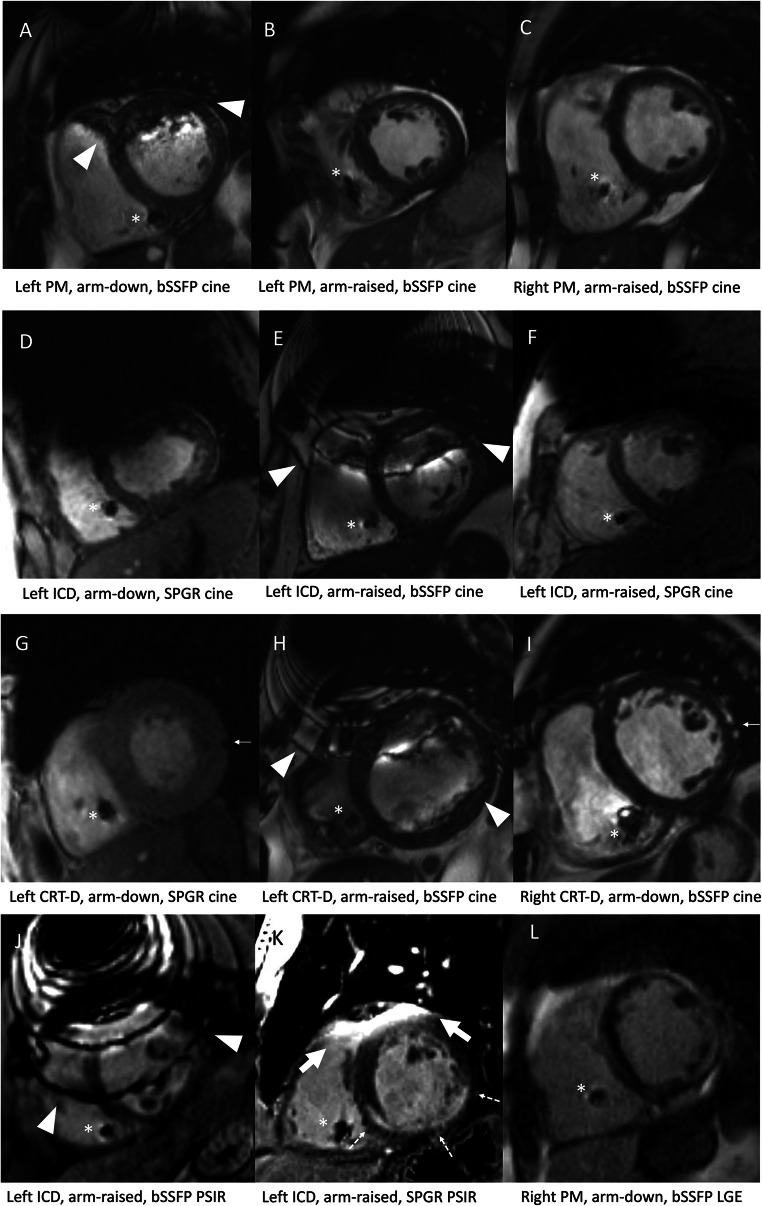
Typical appearances of CIED-related artefacts on CMR images. All images are in short-axis plane. The RV lead-induced artefacts are indicated with an asterisk (A–L). Banding artefacts seen in bSSFP sequences are presented with arrowheads (A, E, H, J). The thick arrow shows hyperintensity artefact, typically seen in SPGR sequences (K). The thin arrow indicates the LV coronary sinus lead-induced artefact (G, I). Generator-induced banding artefact interfering with the myocardium is seen in bSSFP sequences, typically in patients with left-sided generator (A, E, H, J). In SPGR sequences, a large signal-void area induced by the generator is a typical artefact (D, F). Arm-raised scanning position can shift the generator-induced artefacts away from the myocardium (B, F). However, severe artefacts may be present despite the arm-raised scanning position (E, H, J). In patients with left-sided CRT device, LV lead-induced artefact can be obscured by generator-induced artefacts (H). Hyperintensity artefact in LGE imaging could be misinterpreted as positive LGE (K). In image K, the LV anterior wall is affected by hyperintensity artefact, but the subendocardial LGE finding in the LV inferior wall related to ischemic scar is clearly visible. In patients with right-sided generator, the image quality is usually diagnostic (C, I, L). Image L shows patchy intramyocardial and subepicardial LGE in the LV wall extending to the RV wall, related to cardiac sarcoidosis. bSSFP = balanced steady-state free precession; CIED = cardiac implantable electronic device; CMR = cardiac magnetic resonance imaging; CRT-D = cardiac resynchronization therapy; CRT-D = cardiac resynchronization therapy defibrillator; ICD = implantable cardioverter-defibrillator; LGE = late-gadolinium enhancement; LV = left ventricular; PM = pacemaker; PSIR = pPhase-sensitive inversion recovery; RV = right ventricular; SPGR = spoiled gradient echo
